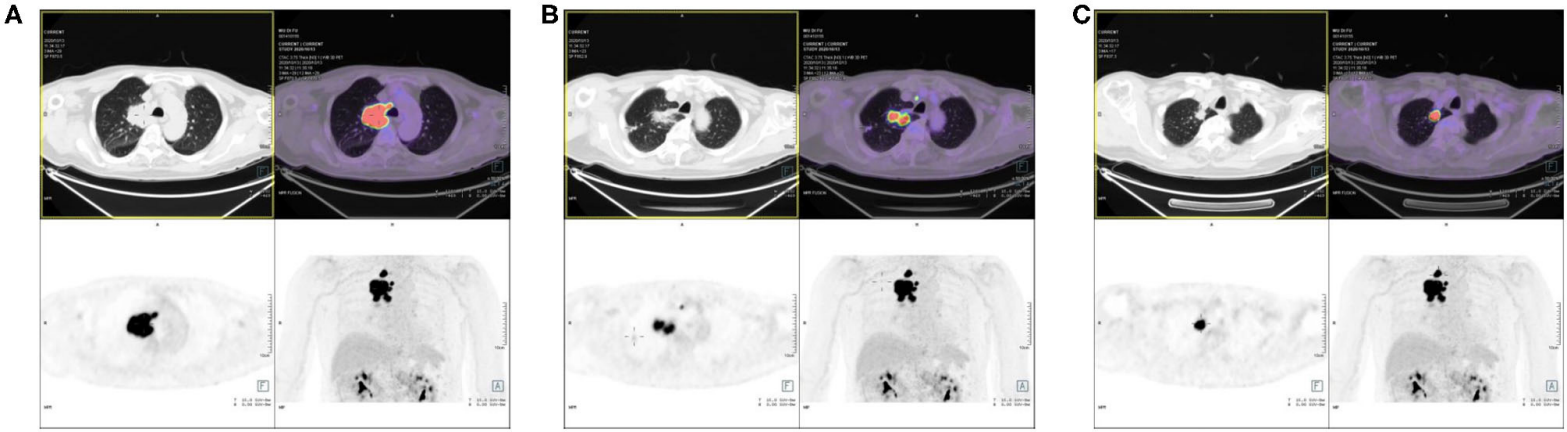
Positron emission tomography (PET)/MRI of the patient. (A) The high metabolic mass in the upper right lobe. (B) The high metabolic nodule at the apex of the right lung. (C) The small nodules in the upper right lobe with traction of the adjacent pleura, with slightly increased metabolism.
